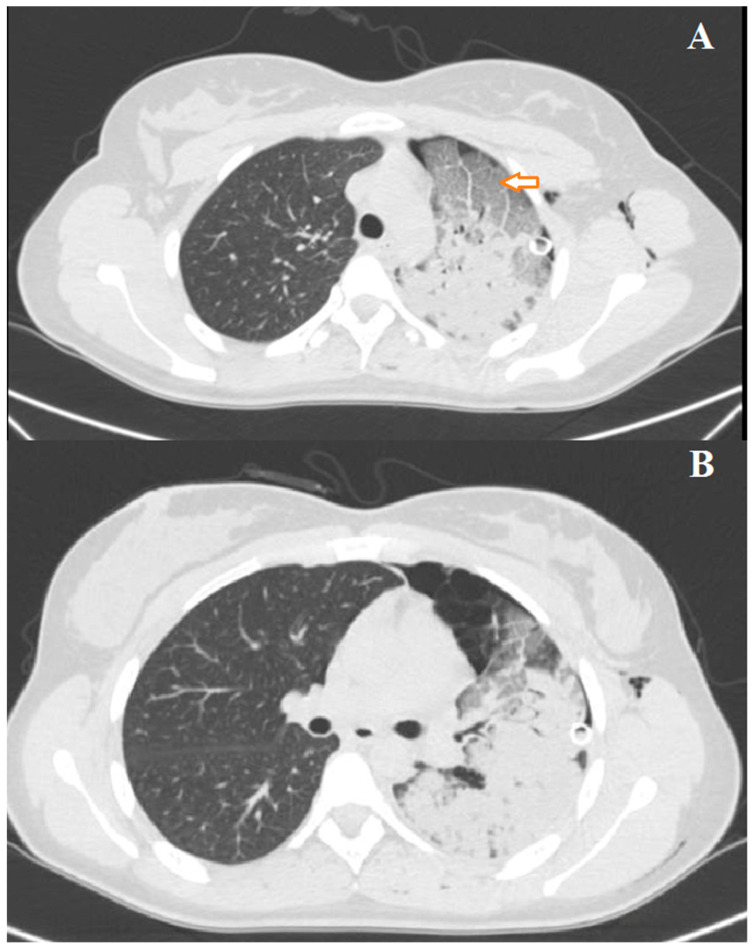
(A,B) Chest CT scan at presentation of left re-expansion edema; the arrow shows alveolar filling.
